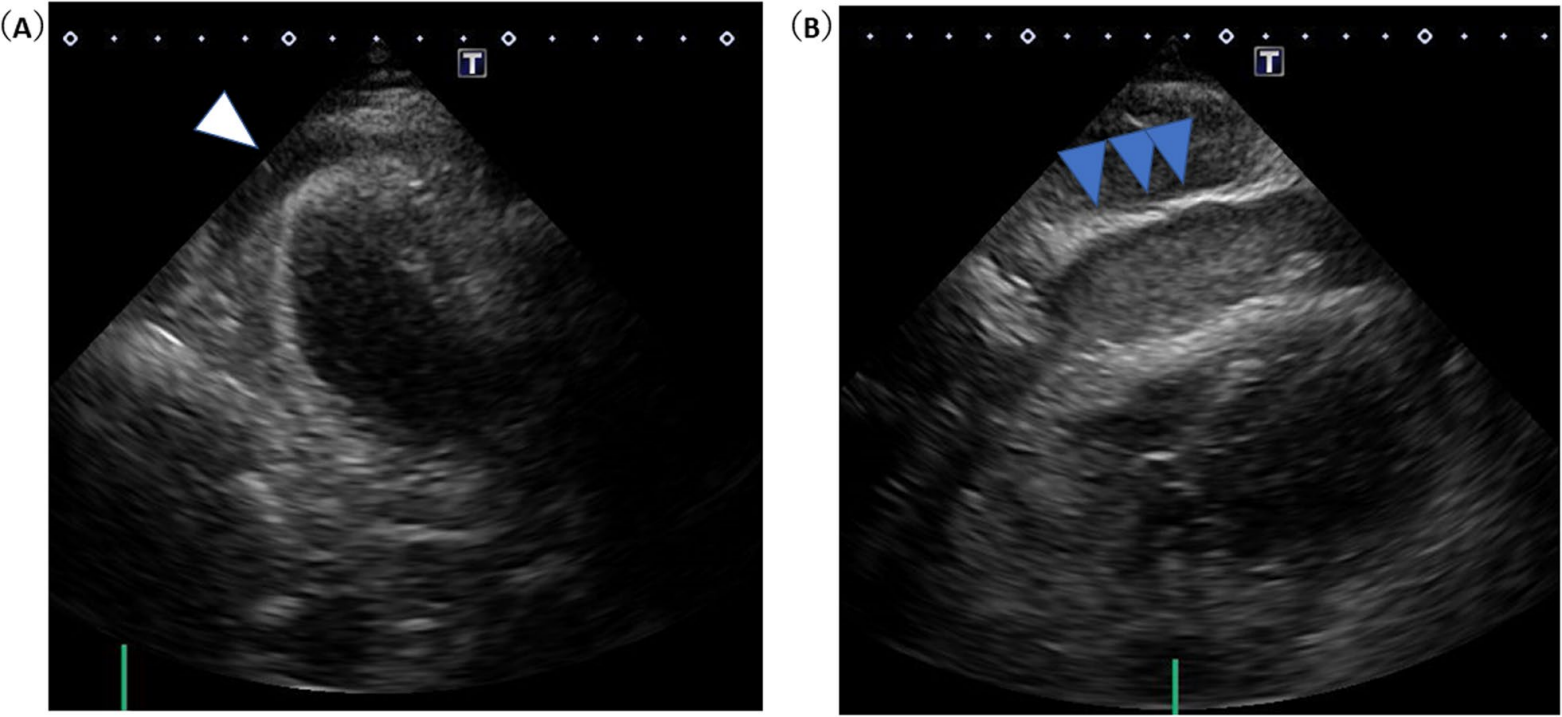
Bedside echocardiography (immediately after transportation). Bedside echocardiography immediately after transfer (A) showed an echo-free space of approximately 5–8 mm, suggesting a clot around the posterior wall (triangle). Twenty-five minutes later (B), a high bright echo-free space was observed that rapidly expanded to more than 30 mm in the anterior right ventricle (triangle).
